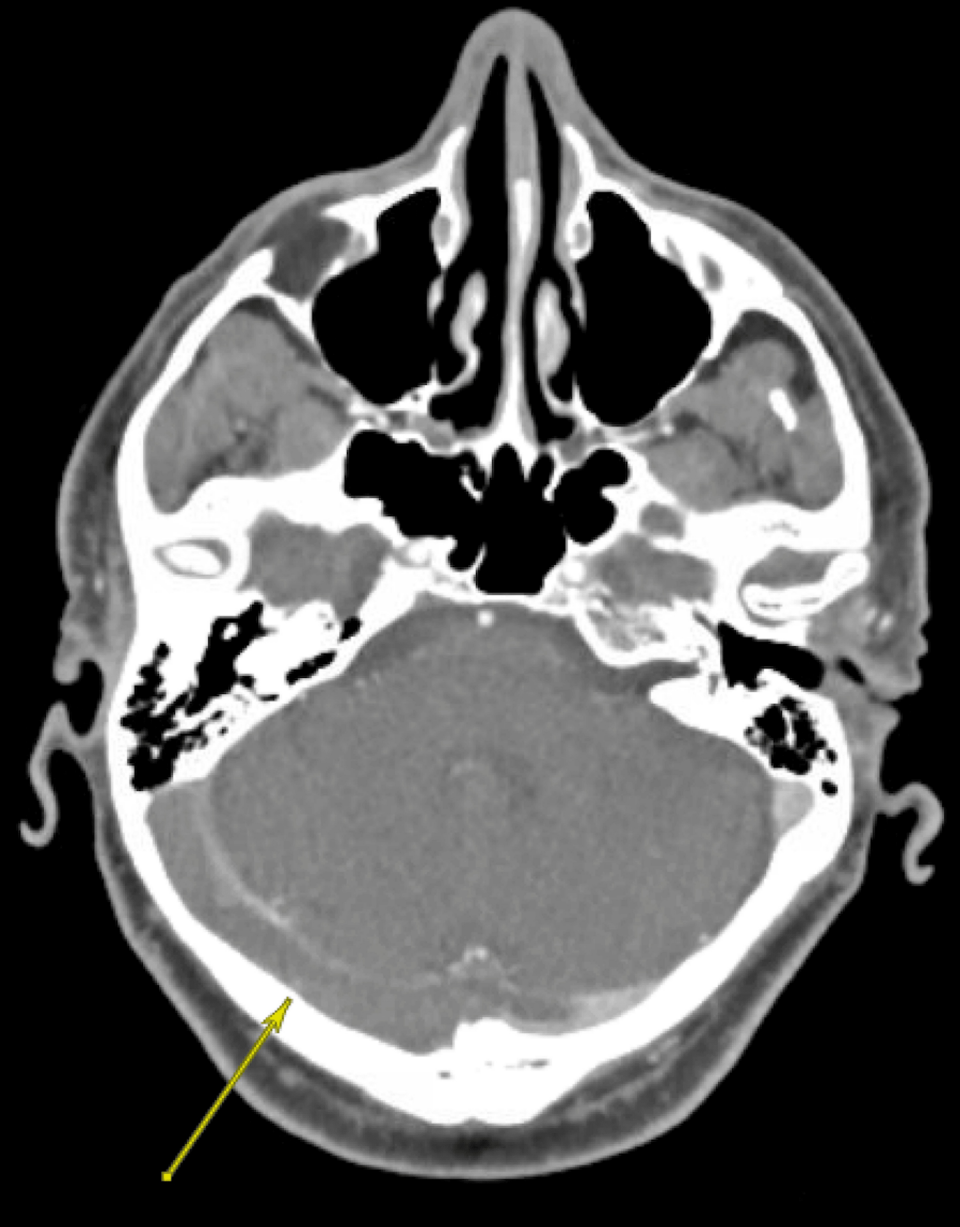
CT venography of the head, axial view, demonstrating filling defects within the right transverse sinus (arrow)
